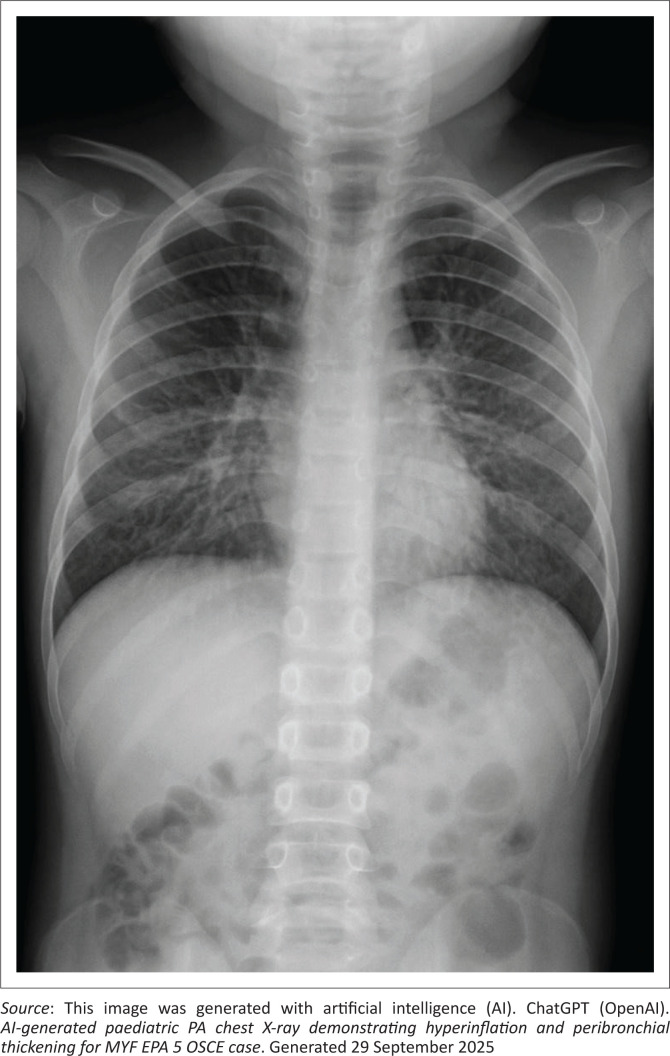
Posteroanterior (PA) chest X-ray.
